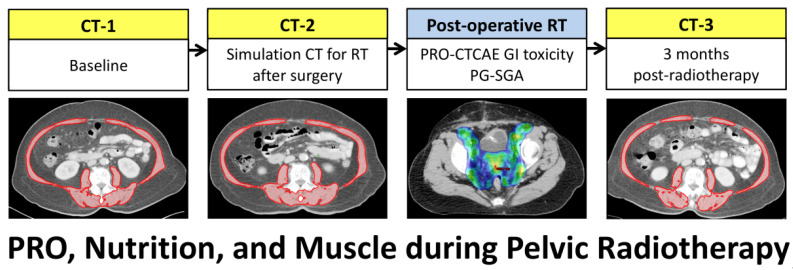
Scheme of CT scans for patients with cervical or endometrial cancer receiving surgery and post-operative pelvic radiotherapy. Skeletal muscle area (red) was measured on a CT slice at the L3 vertebral level. RT, radiotherapy.
